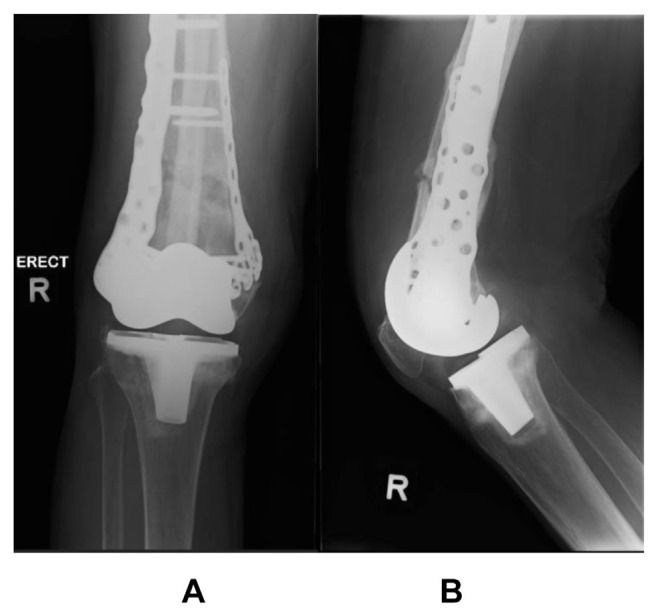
Radiographs at 6 months after surgery.
